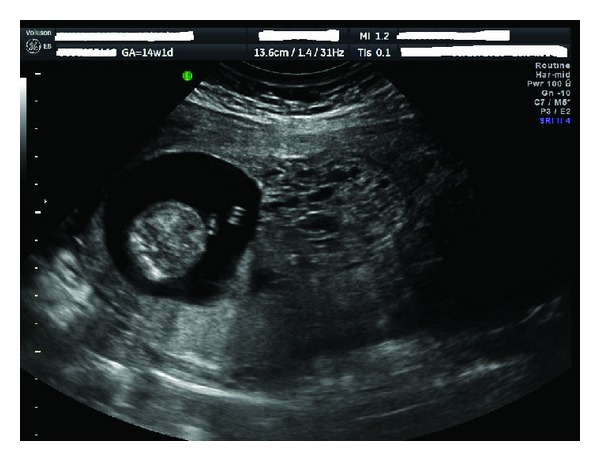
Ultrasound image of twin pregnancy with molar component presenting as a placenta previa and coexisting normal twin at 14 + 1 weeks' gestation.
